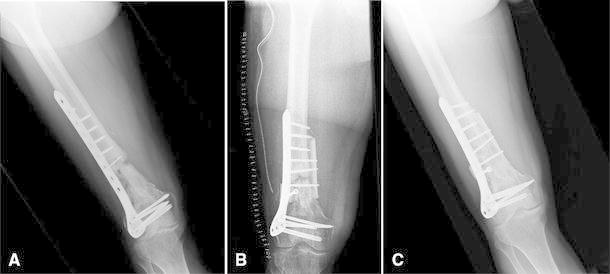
Male 46 years. a Non-union in a complex distal femur fracture. b Post-operative X-ray after revision surgery using homoplastic cortical strut and a new plate. c 1.5 years after second surgery with the AP view radiograph showing fracture healing
